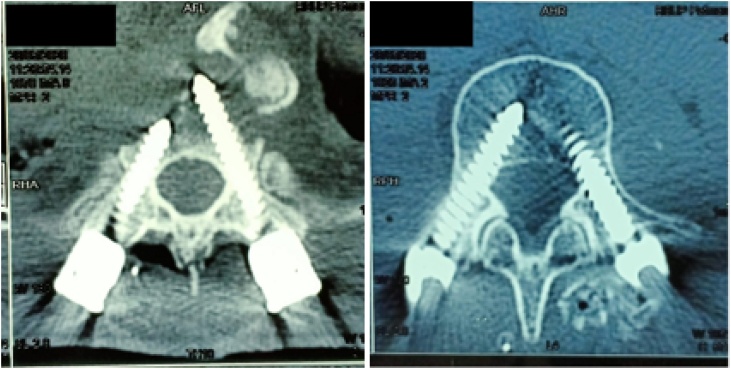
Postoperative CT Scan of the Vertebrae. CT scan showed no screw misplacement, thus excluding screw violation as the cause of postoperative neurological deficit.
